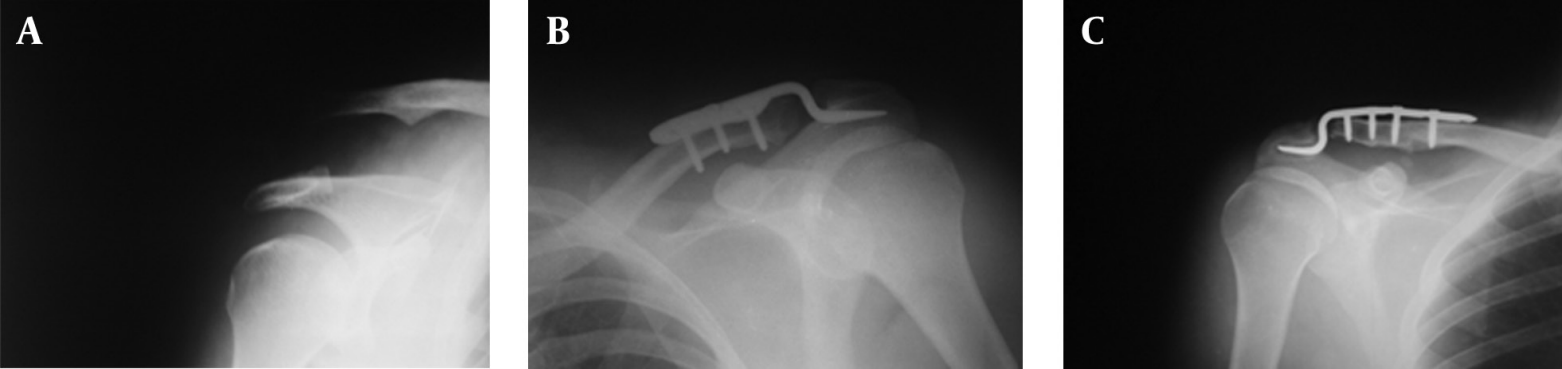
Radiographies A) Acromioclavicular joint dislocation, B) Post operative X-ray, C) Acromial osteolysis
